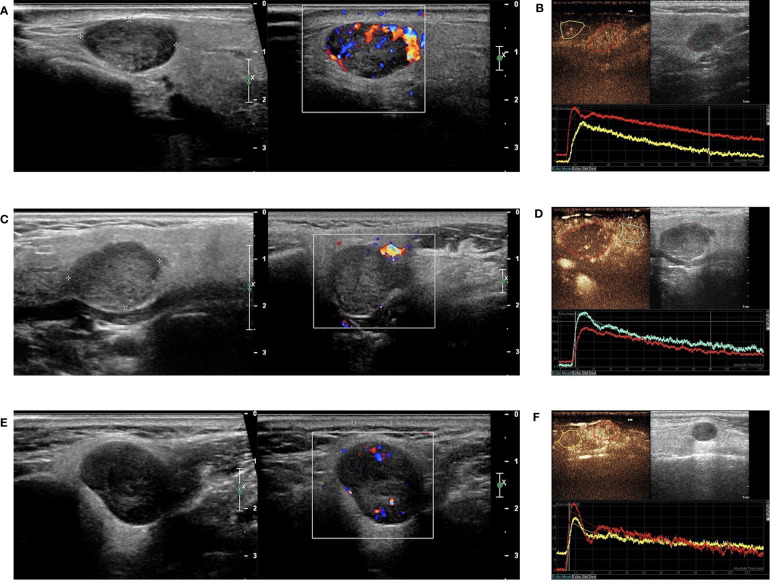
B-mode sonography, Color Doppler sonography (CDs), and dual-imaging of CEUS and gray-scale US with ROI in the remarkable perfusion areas in both the lesions and the surrounding tissues. (A) Hypoechoic and regular masses with well-defined border in the parotid glands of patients with WT, and CDs displayed marked intratumal vascularity in the lesion (grade III); (B) TIC analysis by the QLAB software showed that the red line represented the lesion of WT, while the yellow line represented the surrounding tissues; (C) Hypoechoic and regular masses with a well-defined border in the parotid glands of patients with PA and CDs showing no blood flow signal in the lesion (grade 0); (D) TIC analysis by the QLAB software showing that the red line represented the lesion of PA, while the green line represented the surrounding tissues; (E) hypoechoic and regular masses with a well-defined border in the parotid glands of patients with BCA and CDs showing a small amount of blood flow in the lesion (grade II); (F) TIC analysis by the QLAB software showing that the red line represented the lesion of BCA, while the yellow line represents the surrounding tissues. ROI, region of interest; TIC, time-intensity curve.
